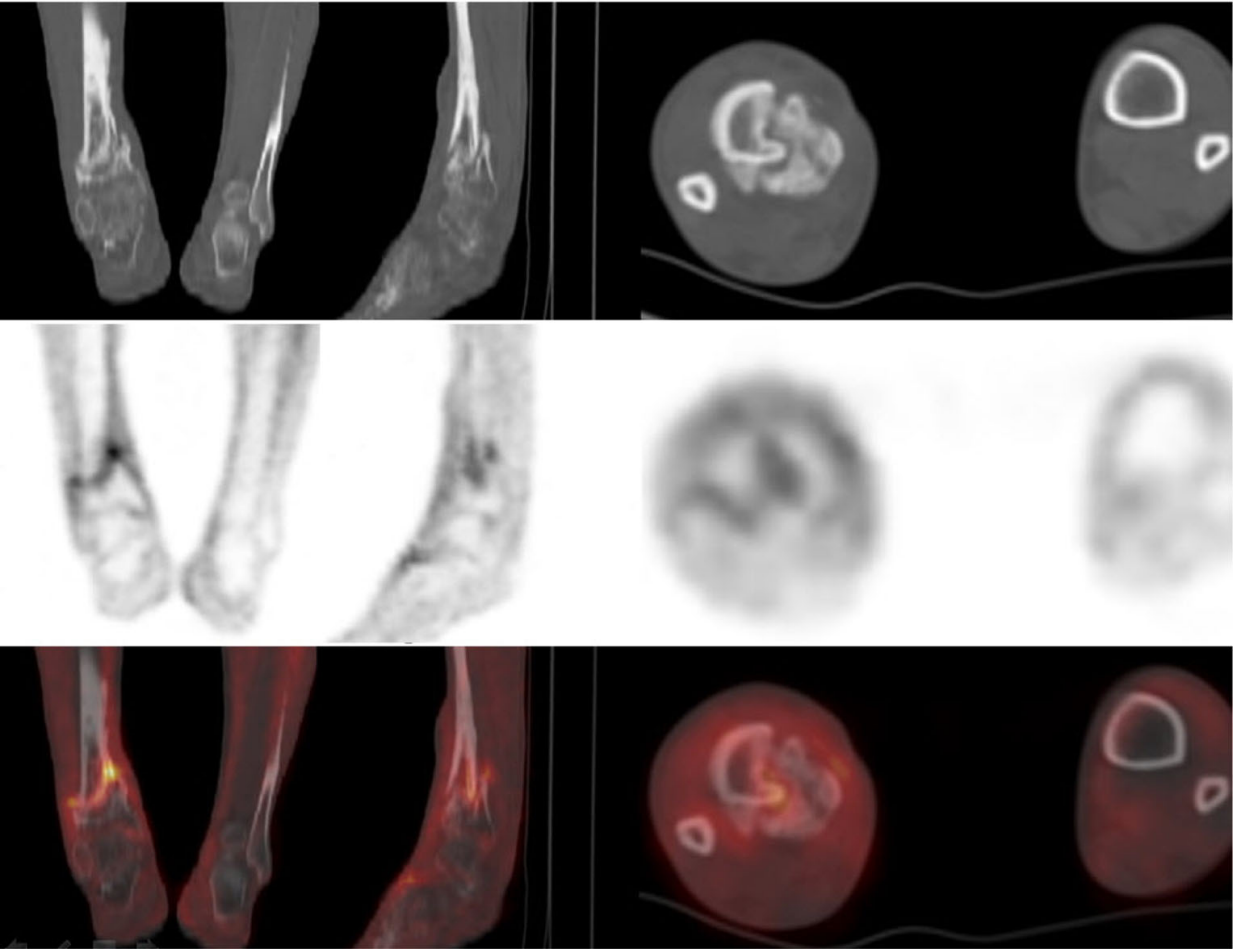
44-year-old patient with oligotrophic non-union fracture of the distal tibia. No complete osseous consolidation was seen for 16 months. [^18^F]FDG uptake was seen along the bone–bone interface of the distal tibia. The [^18^F]FDG uptake was focally increased (SUV_max_ 4.2, SUV_ratio_ 3.1) at the distal part of the proximal bone segment. Only the first and second sets of visual analysis were interpreted as infection of the non-union (true positive). The intraoperative tissue culture was positive
